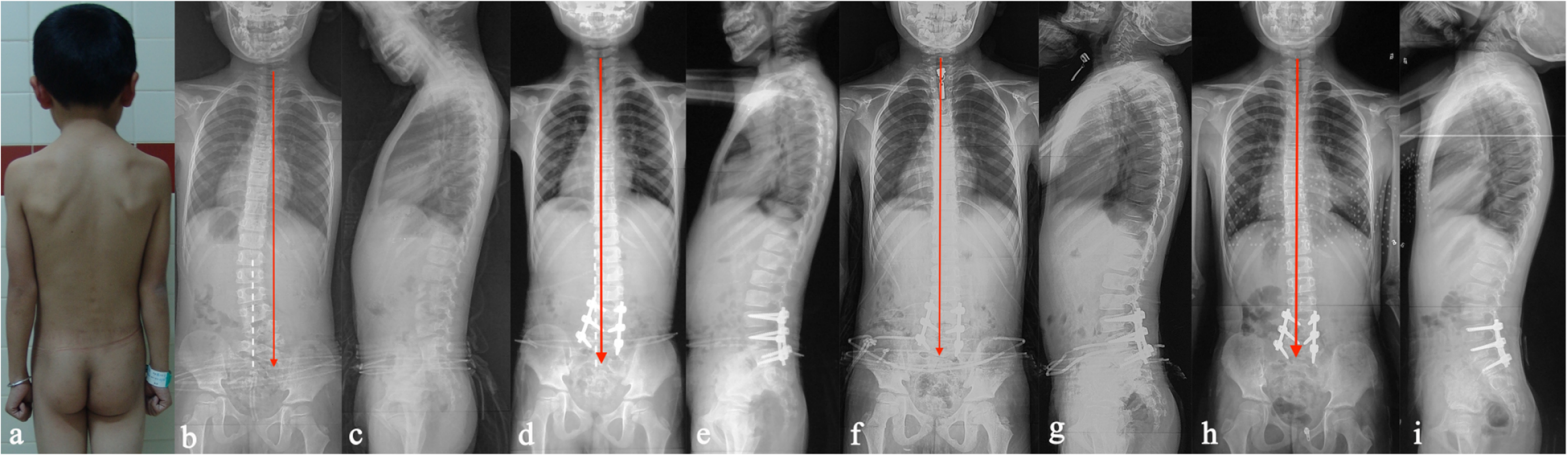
a–c A 6-year-old boy was identified with a lumbosacral hemivertebra located at L5–S1. The patient presented with a long proximal compensatory curve and significant coronal imbalance. d, e Postoperative radiographs showed excellent correction of both the local and compensatory curves and coronal imbalance. f, g The correction was well maintained at the 2-year follow-up. h, i Both the correction of scoliosis and trunk shift were well maintained at the 5-year follow-up
